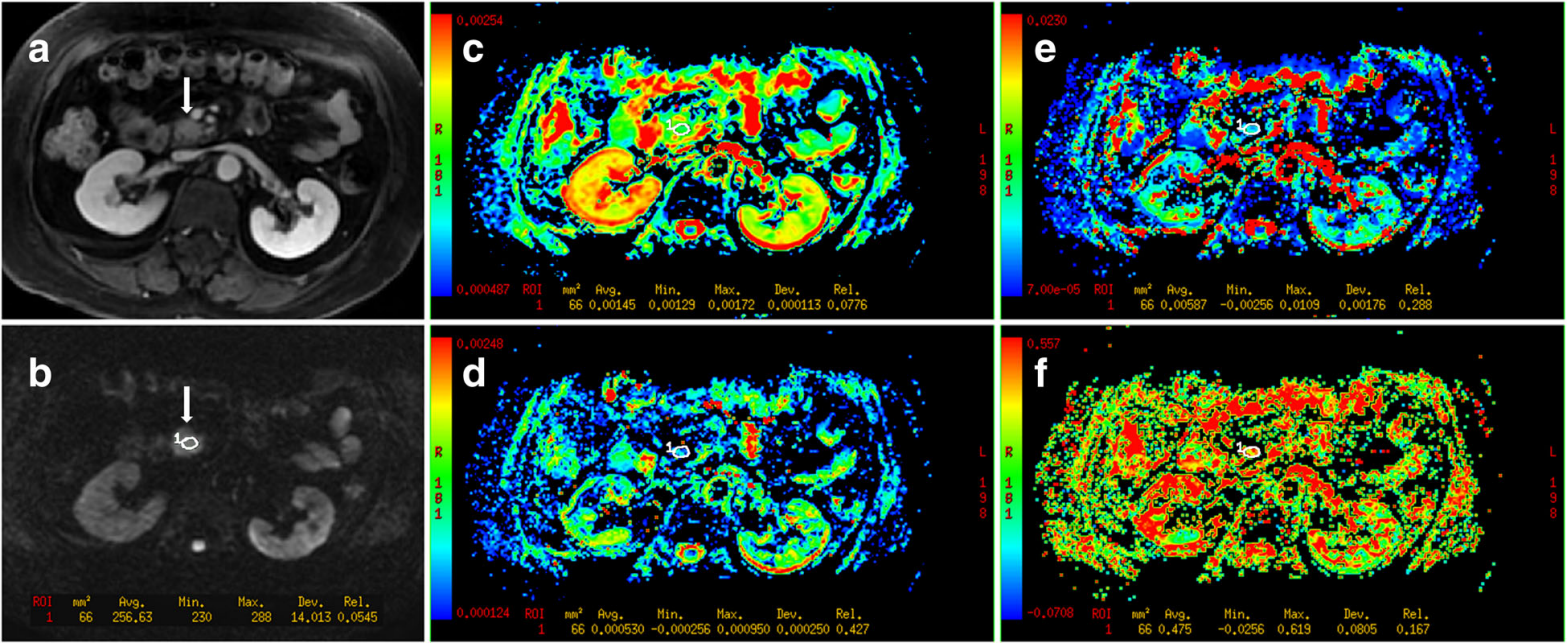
Images from a 67-year-old woman with a moderate differentiated pancreatic ductal adenocarcinoma on the head of the pancreas. (A) Axial contrast-enhanced T1-weighted image shows hypovascularity of the mass (arrow). (B) The nodule is detected on the DW image (b = 1000 s/mm^2^) with clear hyperintensity relative to the remainder of the pancreas (arrow). (C-F) The calculated ADC_total_, ADC_slow_, ADC_fast_ and f -maps, respectively (measurements of the values of ROI were showed in the images)
